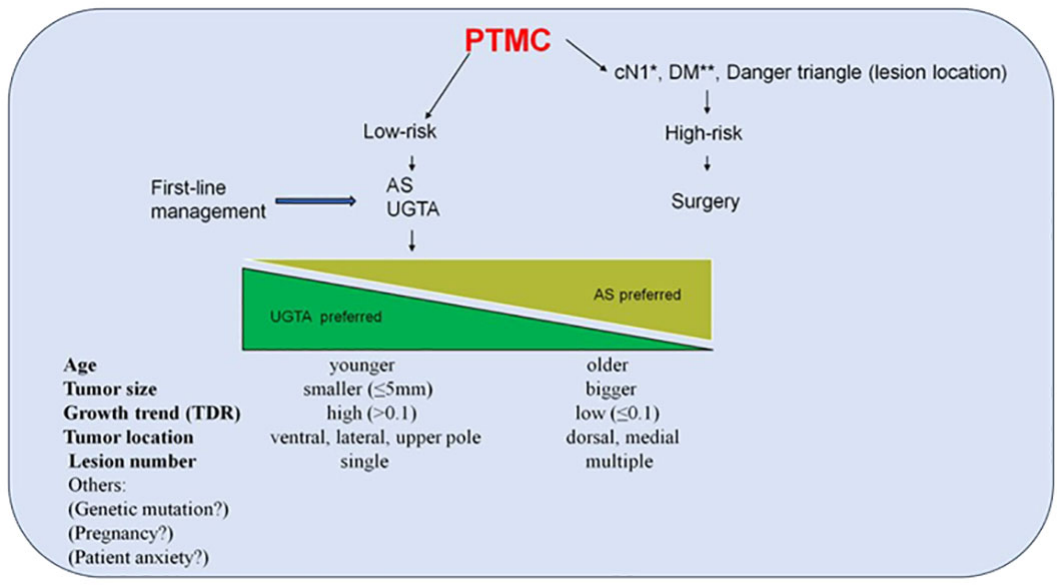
The preliminary framework of PTMC treatment stratification. This framework is a preliminary idea to integrate these three treatments, including surgery, AS and UGTA. UGTA should be preferred over AS if the patient is young, the lesion is small, TDR is high and the location is appropriate. Compared to tumors located on the dorsal or medial side, PTMC located on the ventral or lateral side is more suitable for RFA because it is far from the recurrent laryngeal nerve. For PTC, upper pole location is a risk factor for lateral neck lymph node metastasis, so the PTMC in the upper pole in its early stage should be treated with RFA instead of AS to avoid the possibility of this kind of metastasis. Compared to many small lesions in the thyroid gland, a single lesion is more suitable for RFA, rather than AS. Finally, other factors should be also considered to choose AS or RFA, such as genetic mutations, pregnancy and patient anxiety. *Neck lymph node metastasis confirmed clinically. Distant metastasis. AS Active surveillance; UGTA, Ultrasound-guided thermal ablation; RFA, Radiofrequency ablation.
